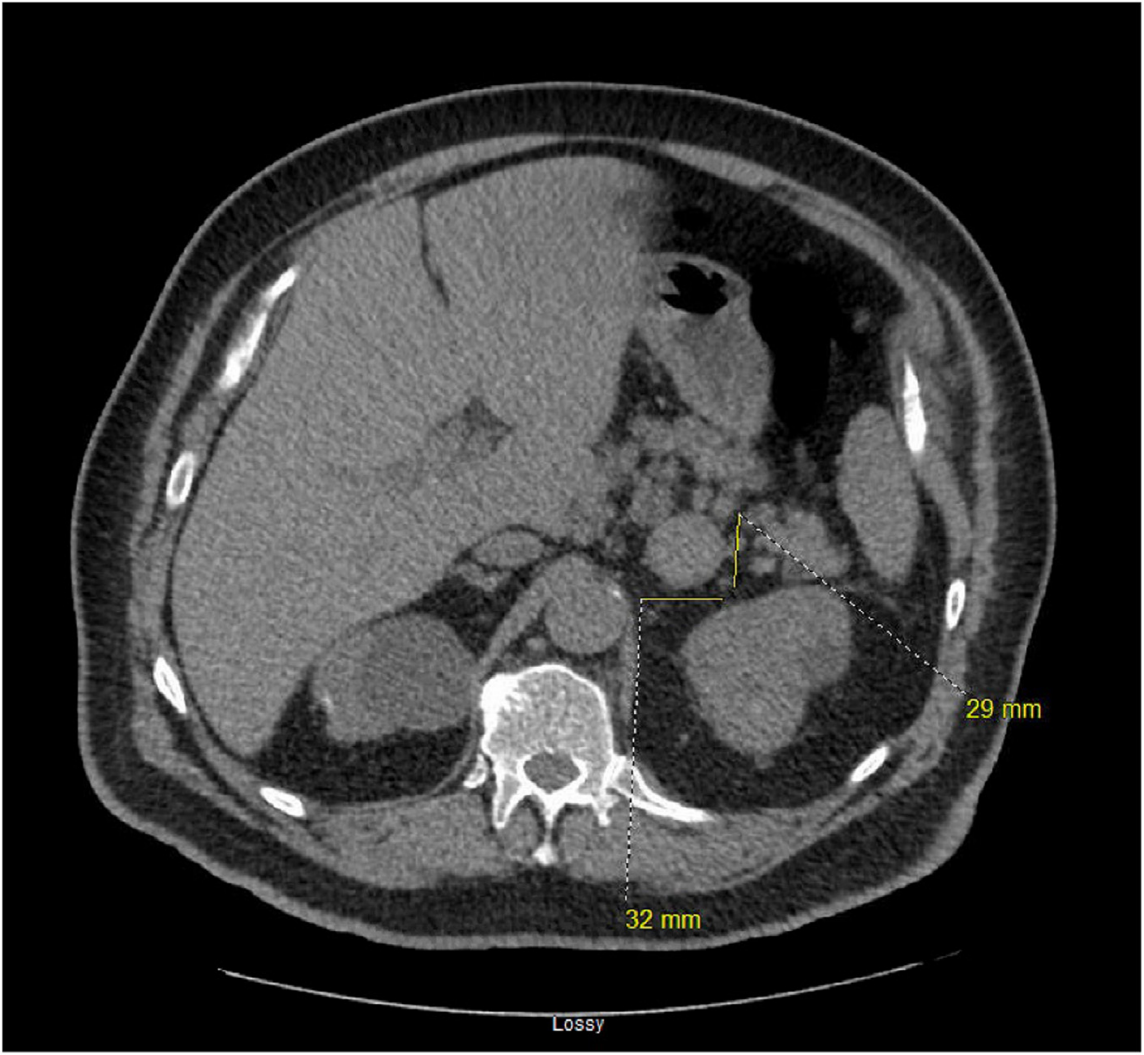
Noncontrast computed tomography (CT) abdomen and pelvis 5 years after initial cancer staging, showing progressive increase in size of the *left* adrenal tumor 32 × 29 mm.
